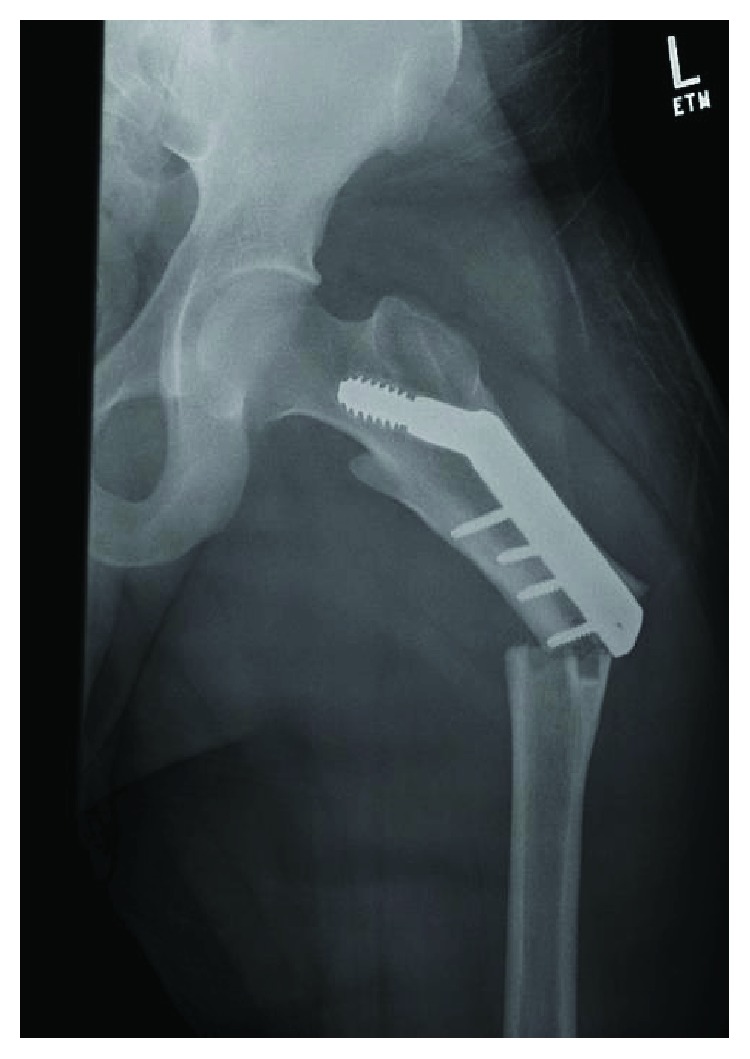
AP view of left hip injury radiographs showing periprosthetic femur fracture with evidence of a potential stress riser at the distal DHS/bone junction, with a significant amount of bony overgrowth of the DHS.
